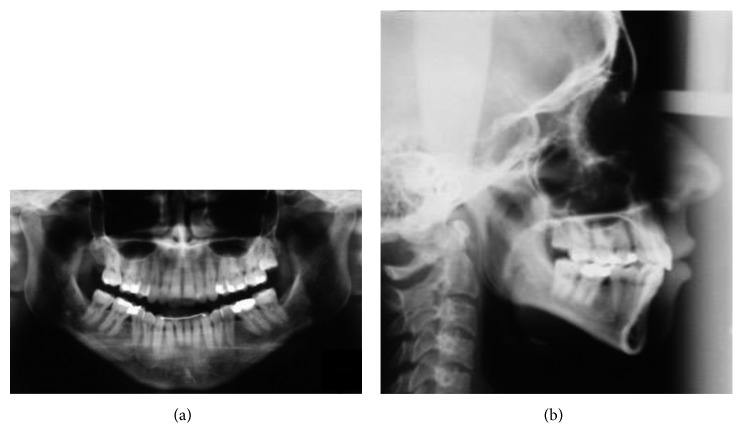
Posttreatment panoramic (a) and lateral (b) radiographs at 22 years, 1 month.
